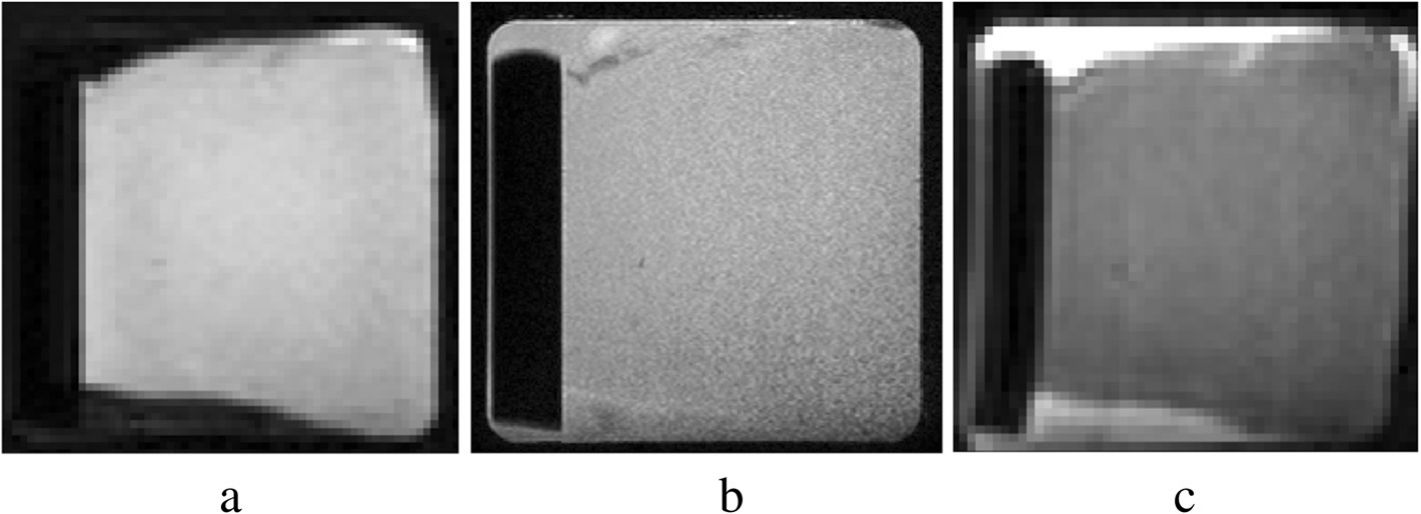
T1-weighted (a), T2-weighted (b) and diffusion weighted with a b-value of 1000 s/mm^2 ^(c) images of one cardiac tissue sample installed in the chamber manufactured in acrylonitrile butadiene styrene by fused plastic deposit.
